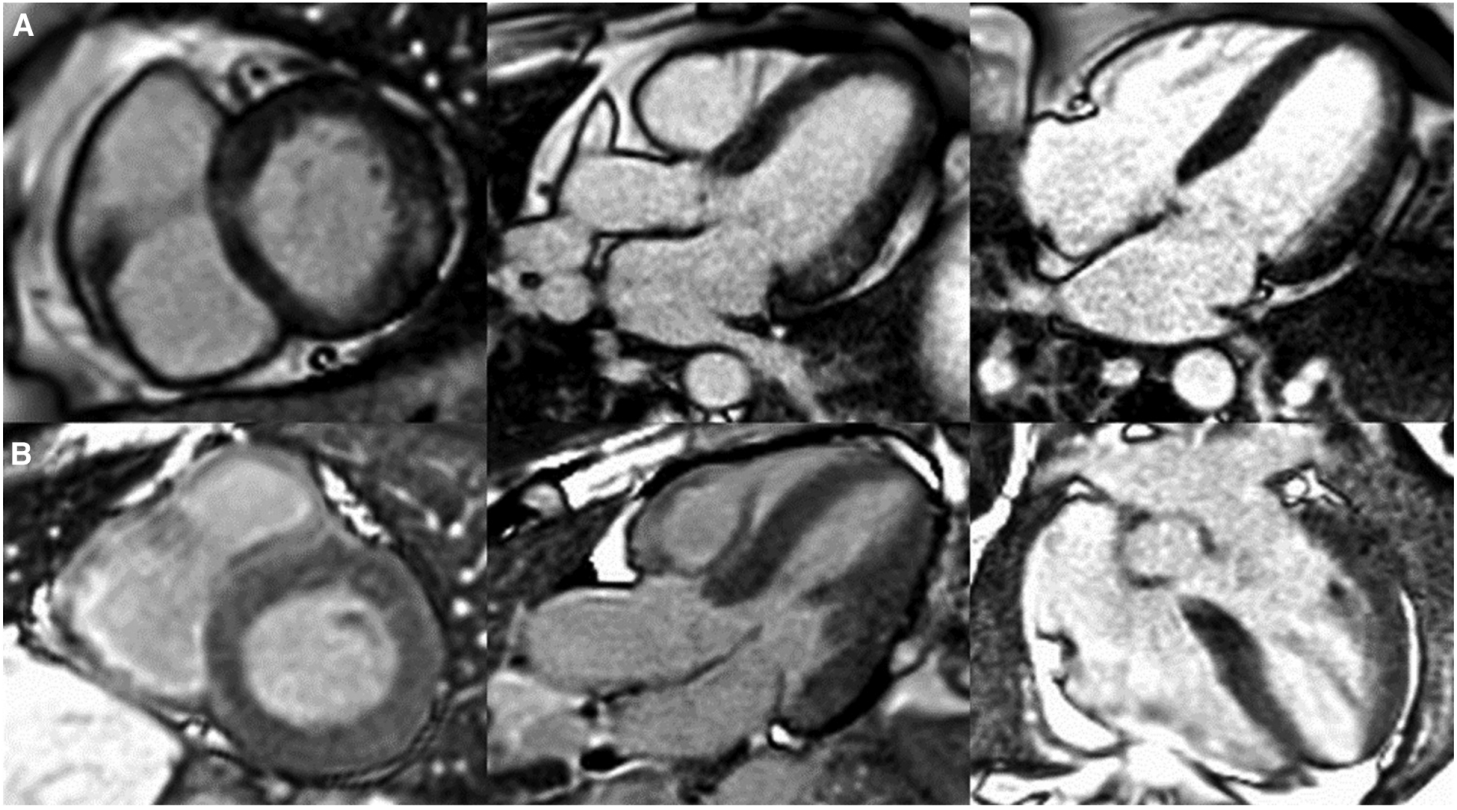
Patterns of cardiac late gadolinium enhancement (LGE) via MRI in Fabry disease. Caption: cardiovascular magnetic resonance LGE images captured using phase sensitive inversion recovery sequences with motion correction. Panel (A), left to right: basal short axis, 3 chamber and 4 chamber views depicting pathognomonic basal inferolateral wall fibrosis in a female patient (60 years old) with no left ventricular hypertrophy (maximal wall thickness = 7 mm). Panel (B), left to right: basal short axis, 3 chamber and 4 chamber views depicting lack of LGE, therefore fibrosis in a male Fabry patient (51 years old) with left ventricular hypertrophy (maximal wall thickness = 17 mm in the basal antero-septum).
